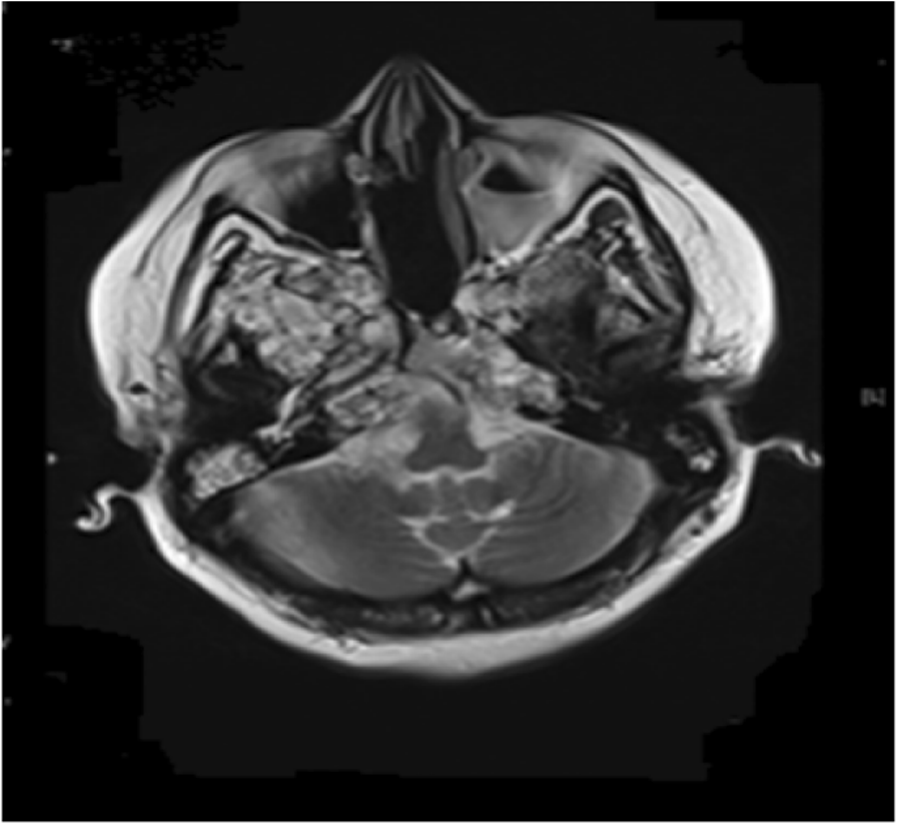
Magnetic resonance image of patient 3 treated with imatinib (progressive disease 25 months)
